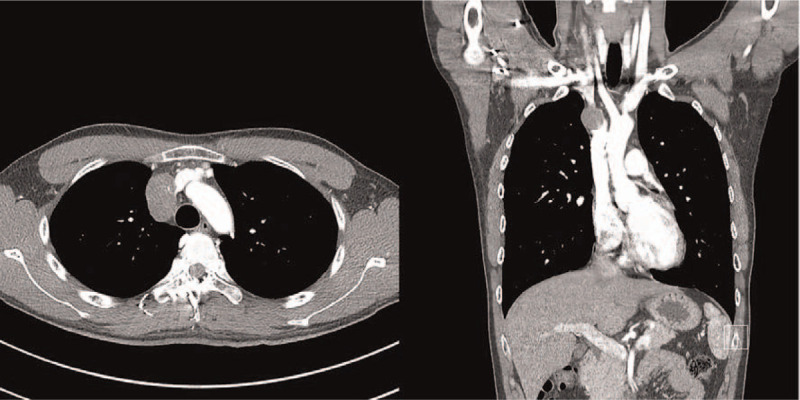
Computed tomography shows a 3.8 × 2.5 cm sized, well-defined mass with smooth margins, tiny punctate calcifications and mild contrast enhancement of the intrathoracic great vessel.
